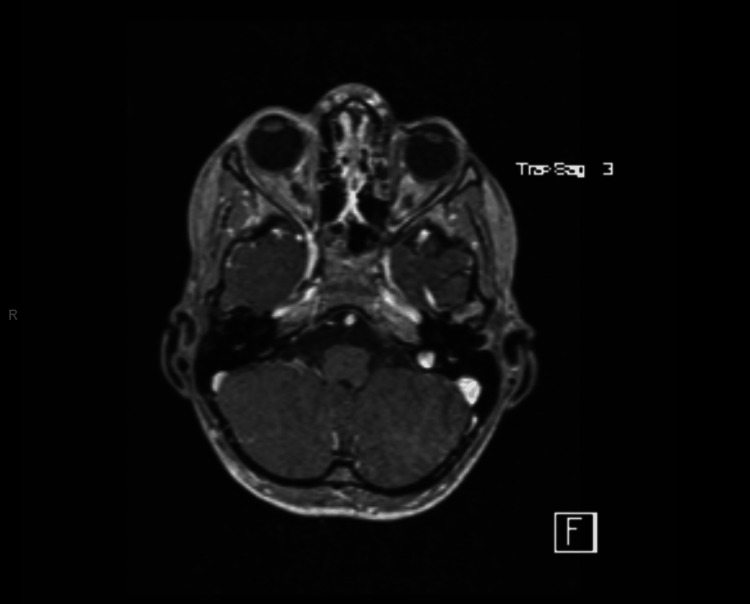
Axial T2-weighted MRI images of the posterior fossa and internal auditory canals showing normal labyrinthine structures without enhancement or mass lesion.
